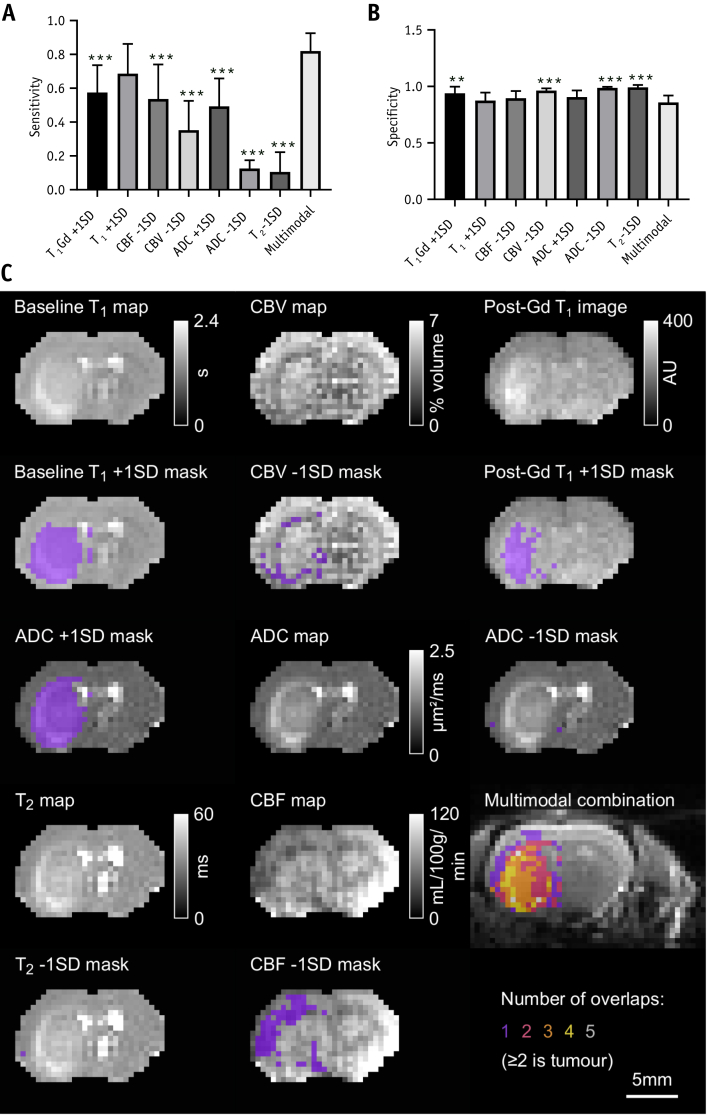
Comparisons with individual modalities and example MRI scans. (A) Sensitivity and (B) specificity of tumor detection using individual MRI metrics, as well as the multimodal MRI method. *P* < .01; P* < .001 with respect to multimodal MRI; analysis of variance followed by Dunnet’s post hoc test. (C) Example MRI maps and images, alongside overlays of the same map or image with the relevant threshold mask. The multimodal combination map shows the final spatial localization of the tumor by the multimodal MRI method (the multimodal MRI tumor definition is the area where at least 2 modalities have identified an abnormality). *Abbreviations*: ADC = apparent diffusion coefficient; CBF = cerebral blood flow; CBV = cerebral blood volume; MRI = magnetic resonance imaging.
